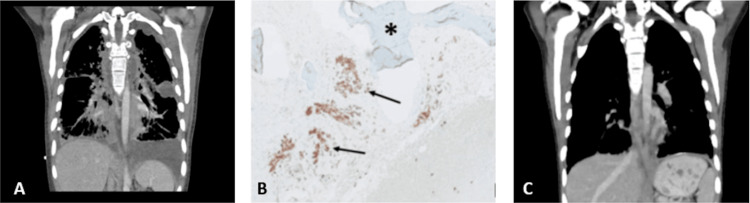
Pulmonary and bone marrow metastases (A) CT Chest with diffuse nodular parenchymal disease and bilateral pleural effusions; (B) Bone marrow biopsy showing  bone trabeculae (*) and cells that are positive for Olig2 (arrows); (C) CT Chest with an improvement of nodular parenchymal disease and improvement of bilateral pleural effusions
